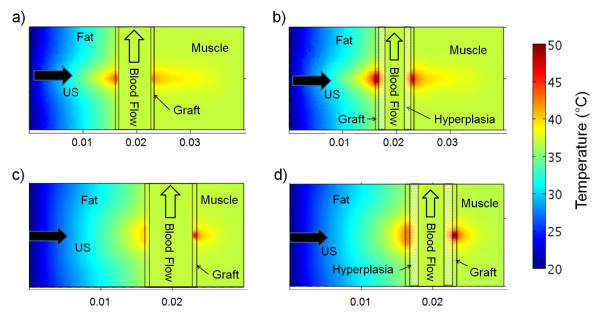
Temperature in the graft models, in the central plane aligned with the graft length, after 30 seconds of ultrasound exposure. 0.6 W of acoustic power was applied to the 1.5-MHz models with (a) no hyperplasia and (b) 1.2-mm of hyperplasia. 0.375 W was applied to the 3.2-MHz models with (c) no hyperplasia and (d) 1.2-mm of hyperplasia. With hyperplasia, 50°C was reached within the graft material for both transducers.
